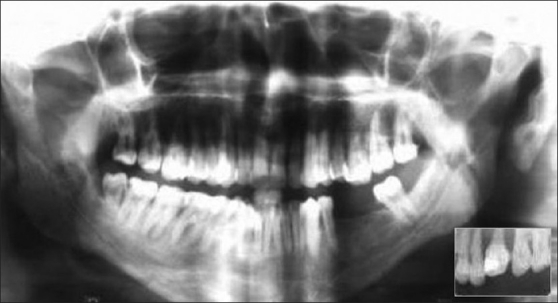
Orthopantamograph and IOPA radiograph showing bone loss in relation to 16 and 17
